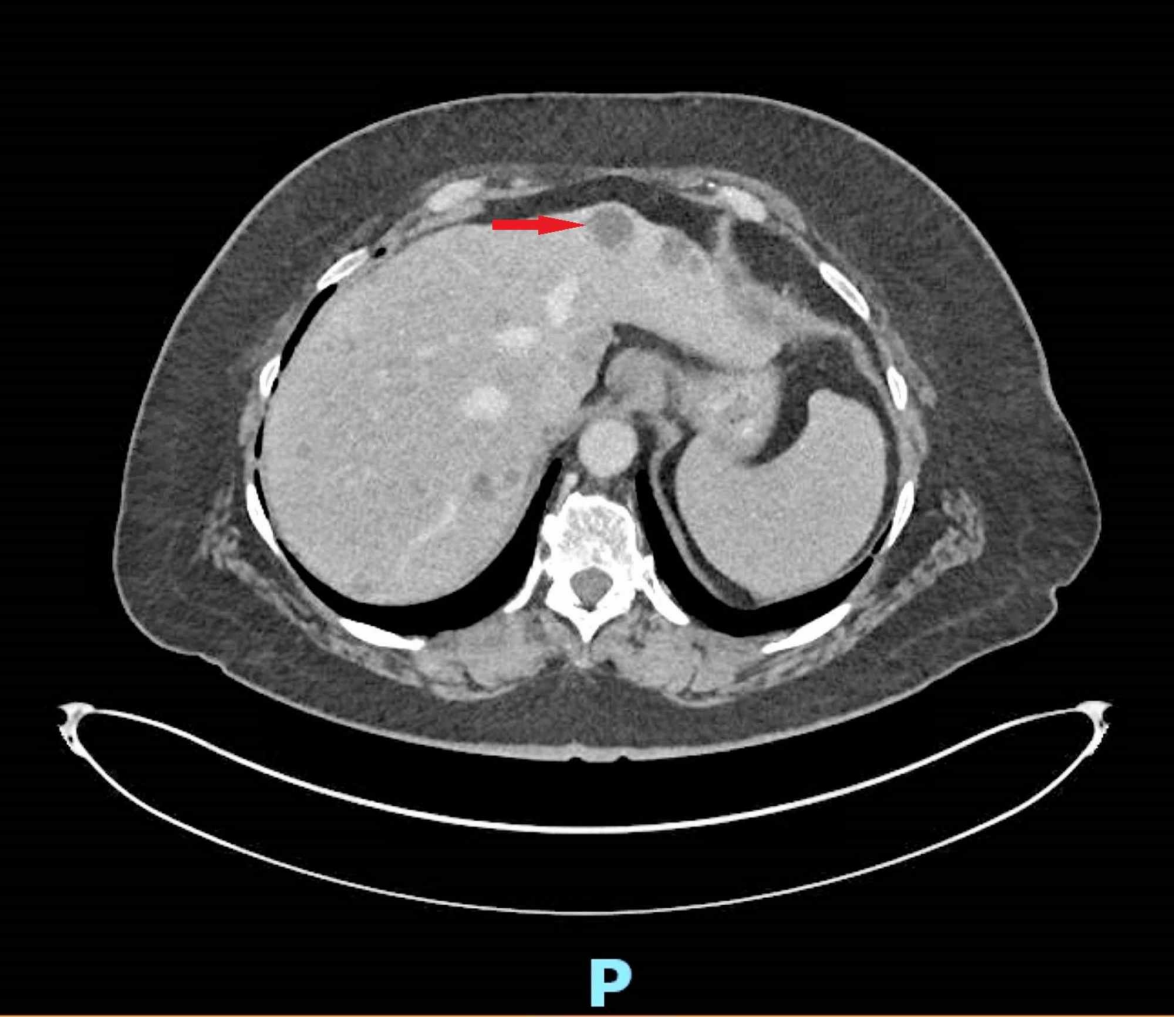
CT scan of the abdomen and pelvis with contrast showing hepatic lesions concerning for metastatic disease.
